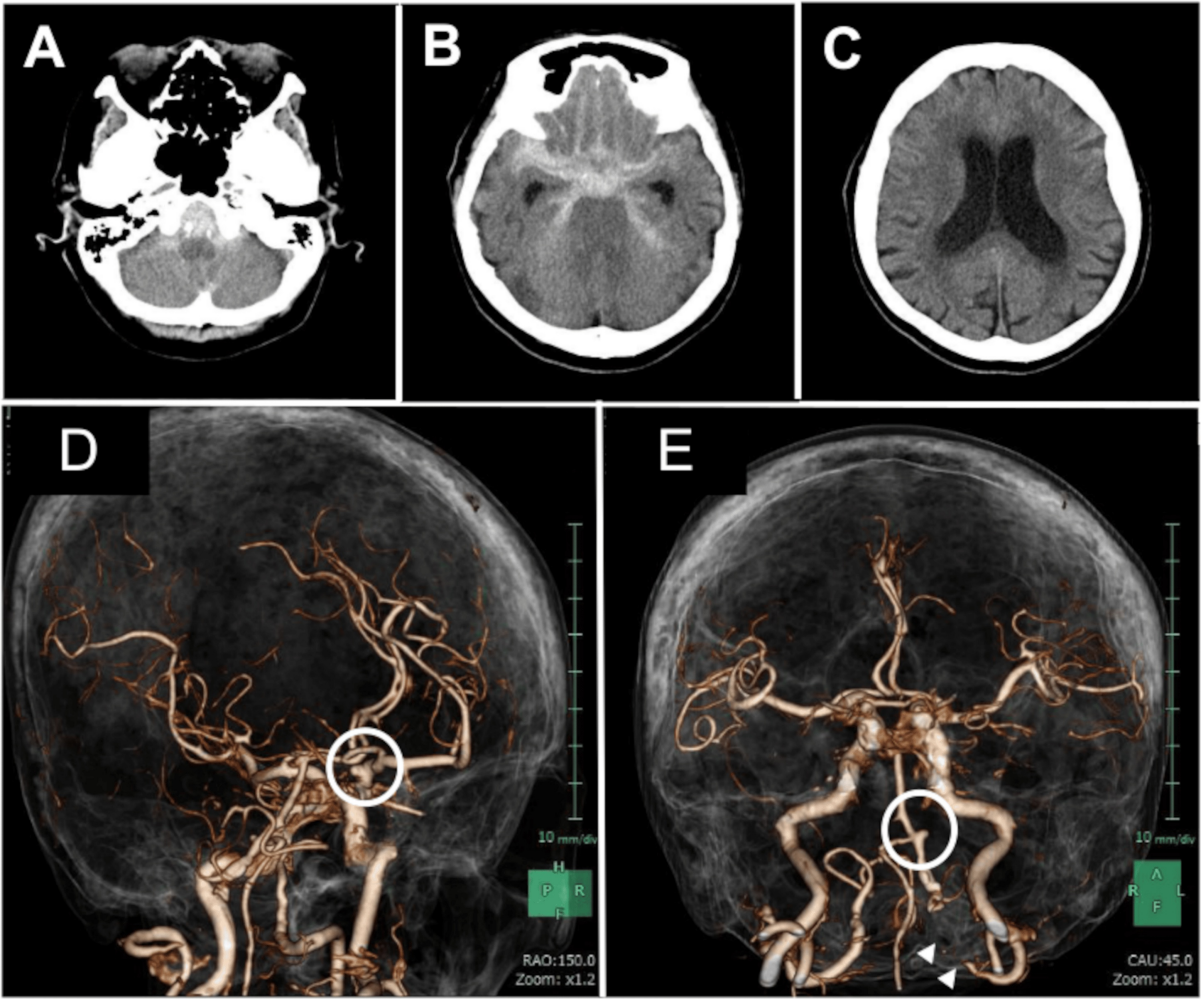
Preoperative computed tomography (CT) and CT angiography. (A, B) Subarachnoid hemorrhage (SAH) is identified in the posterior cranial fossa and basal cisterns, predominantly on the right side. (C) Concurrent acute hydrocephalus is observed. (D) A right internal carotid-posterior communicating artery aneurysm is observed (white circle). (E) A left saccular intracranial vertebral artery aneurysm is simultaneously identified on CT angiography (white circle). The left vertebral artery is occluded (white arrowheads).
